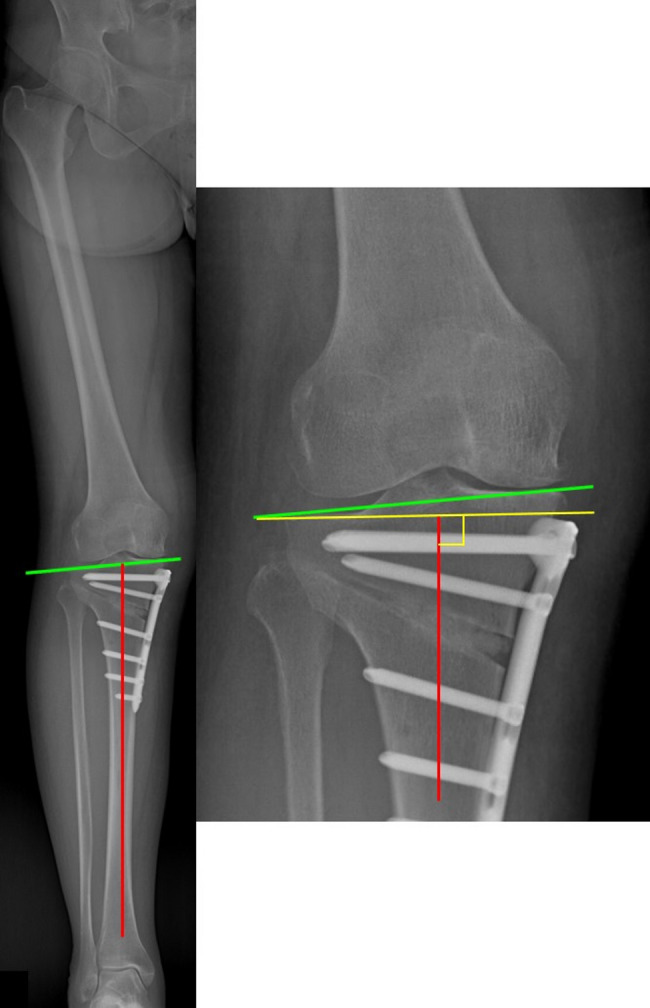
Representative long-standing hip-to-ankle radiograph and magnified knee image demonstrating the knee joint orientation, which was defined as the angle between the tibial articular line (green line) and the horizontal reference line (yellow line). The red line indicates perpendicular line to the ground
